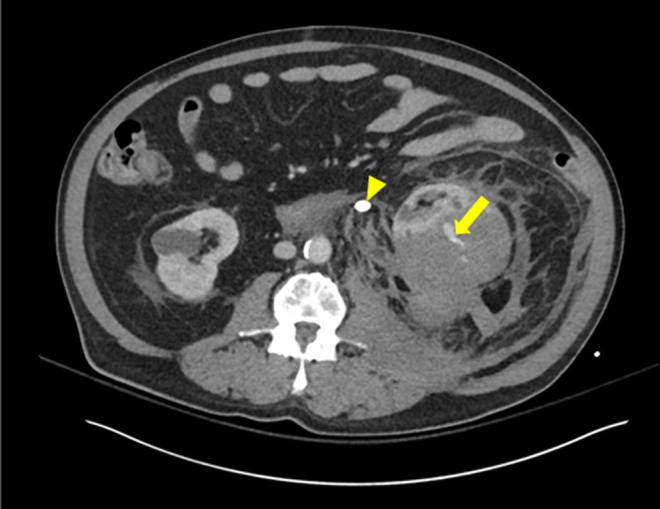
CT scan of abdomen and pelvis with IV contrast axial section showing perinephric hematoma, which deformed and compressed the kidney anteriorly. *Arrow* points to an area of active extravasation of contrast within the hematoma. *Arrow head* points to 1.1 cm obstructive proximal ureteral stone. Marked left perinephric stranding was noted. CT, computed tomography.
